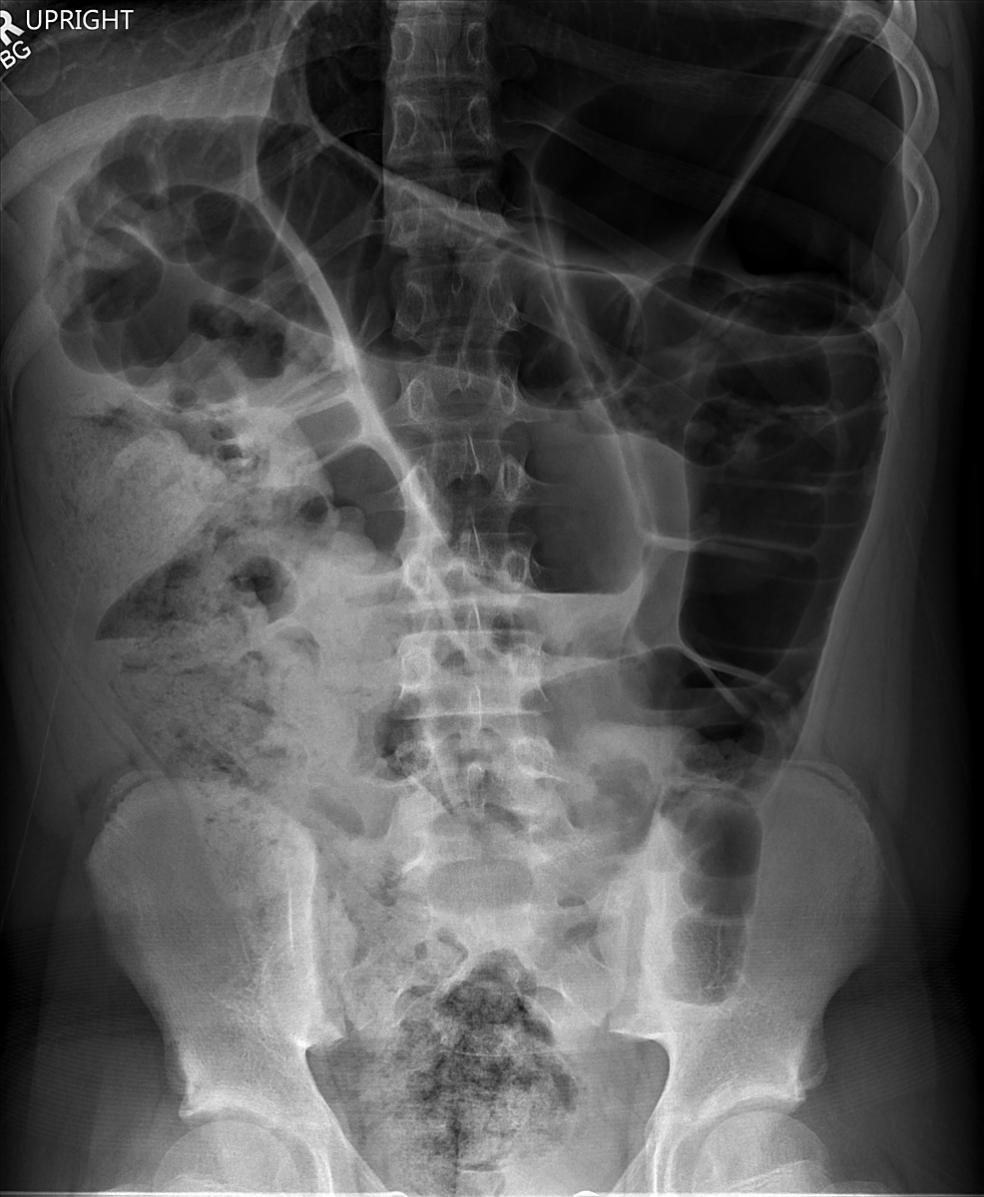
Abdominal X-ray at admission showing dilation, suggesting sigmoid volvulus. There is also moderate fecalization of the distal colon.
